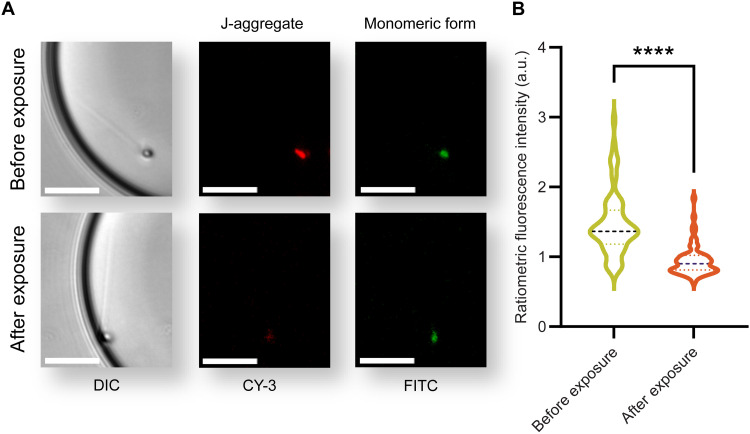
Sperm MMP change upon ultrasound exposure. (A) Representative images of an example individual sperm labeled by JC-1 dye to evaluate the fluorescence intensity of red J-aggregates and green monomers before and after exposure using CY3 and fluorescein isothiocyanate (FITC) filters, respectively. The images were contrast-adjusted for clarity. Scale bars, 20 μm. (B) Comparison between the ratiometric fluorescence intensity of red J-aggregate to green monomers before and after exposure to ultrasound (*n* = 58, from three independent experiments using three biologically independent human samples). Values are reported as means ± SD (table S7). Statistical significance was determined using paired *t* test (*P* ≤ 0.0001). DIC, differential interference contrast; a.u., arbitrary units.
